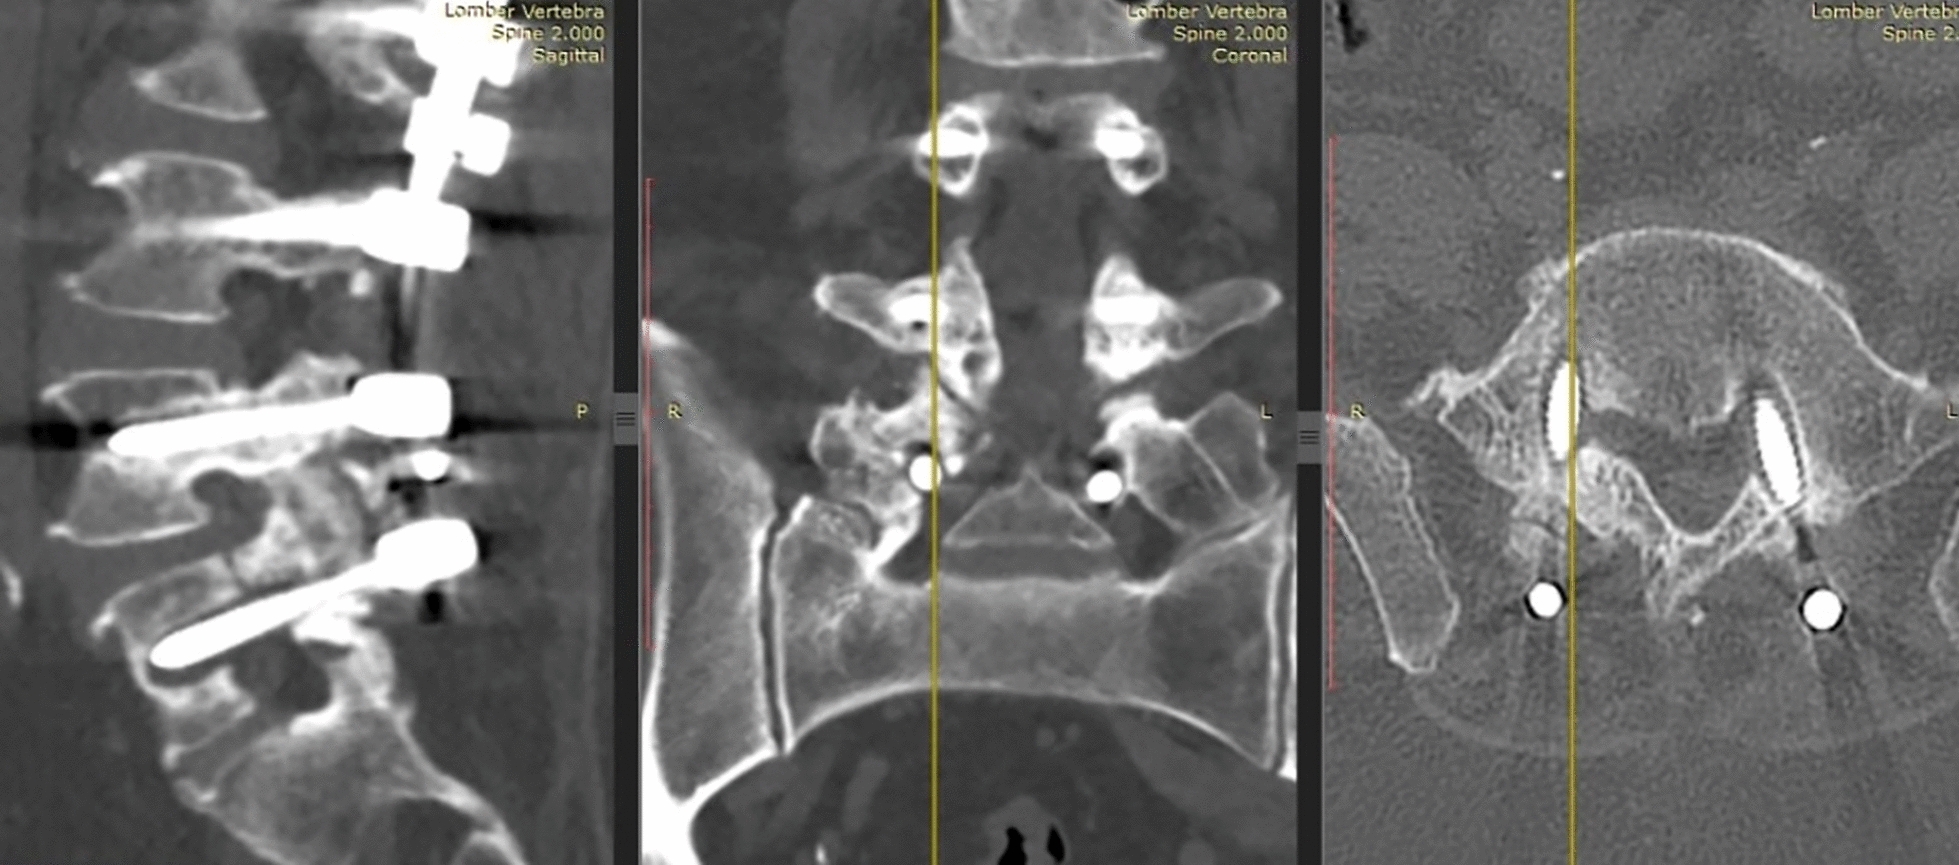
Axial, coronal and sagittal views demonstrate screw loosening on CT scans
